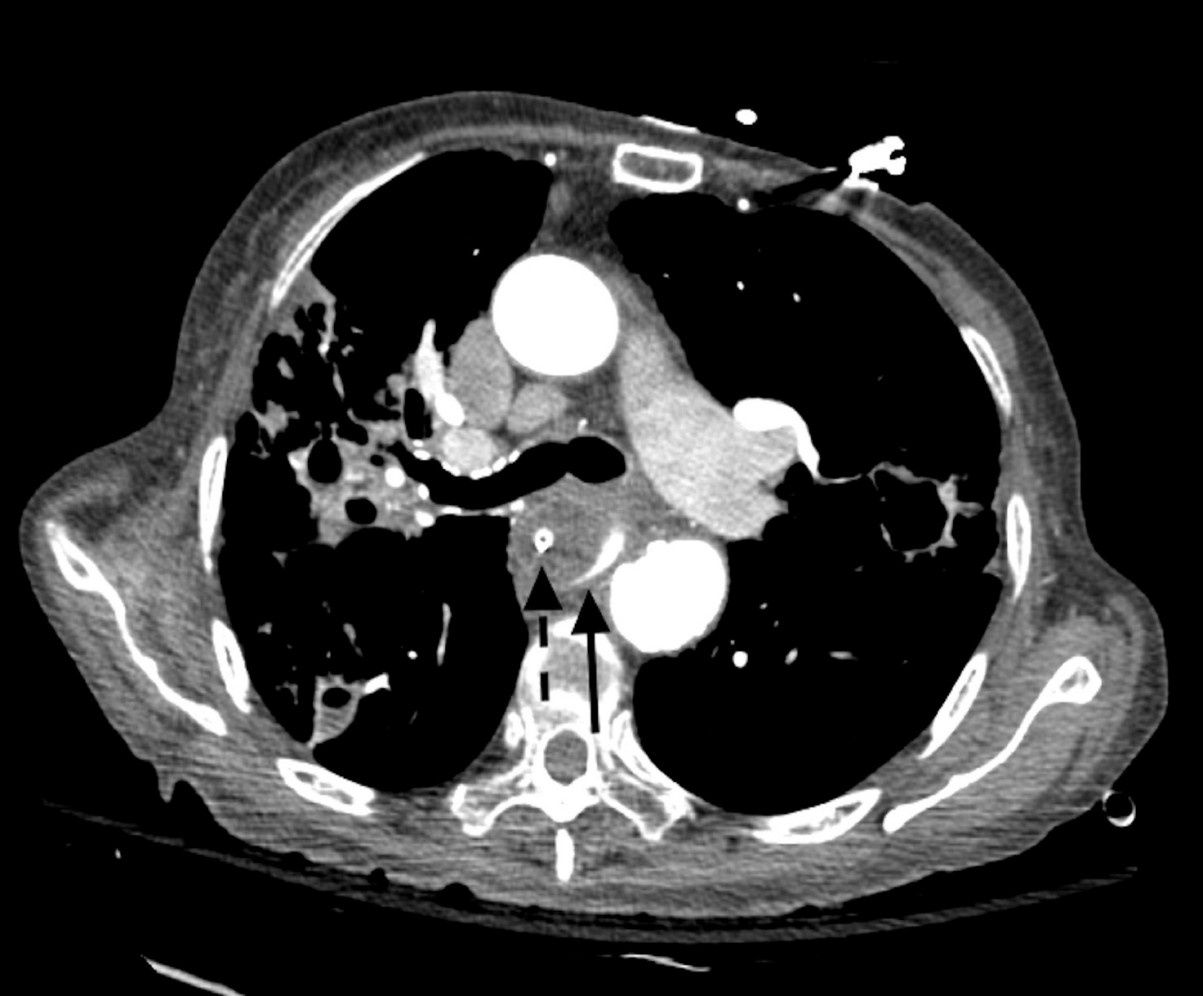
Computed tomography of the chest with contrast filling the esophagus (solid arrow) and adjacent oral gastric tube (dashed arrow).
